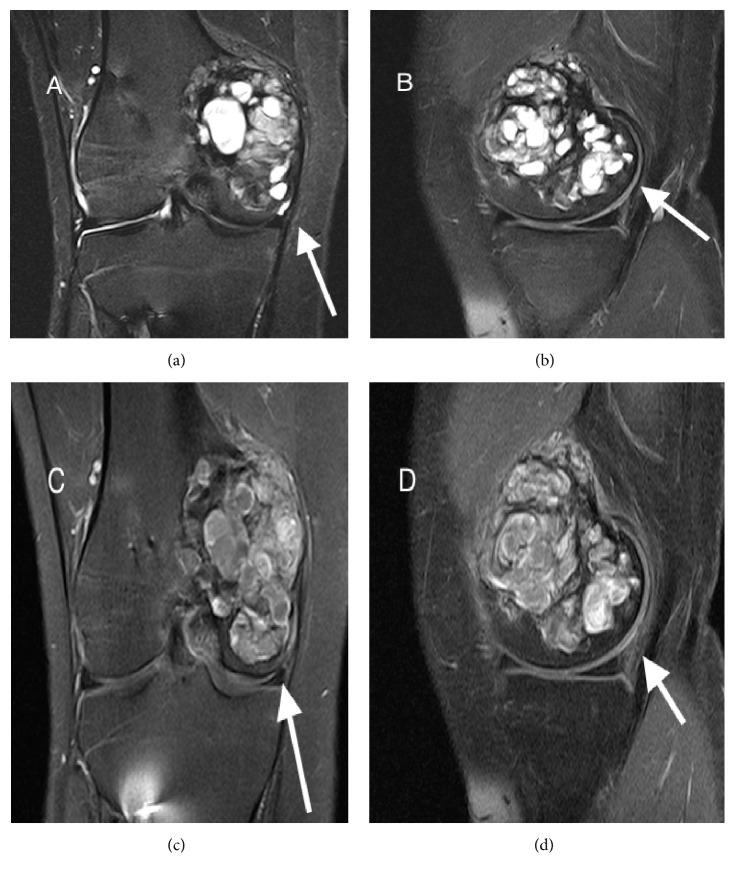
MRI of the right knee 4 years after initial presentation demonstrates an expansile multilobulated mass in the medial femoral condyle. (a) Coronal T2 turbo spin echo (TSE) short tau inversion recovery (STIR), (b) sagittal T2 TSE fat saturation, and (c) coronal and (d) sagittal T1 TSE fat saturation images demonstrating marked interval increase in size of a T1 and T2 heterogeneous multilobulated, expansive osseous lesion, as indicated by the arrows, extending from the posterior distal medial femoral metaphysis to the medial femoral epiphysis. Within this mass are multiple fluid filled spaces with fluid-fluid levels suggestive of intralesional secondary aneurysmal bone cyst formation.
